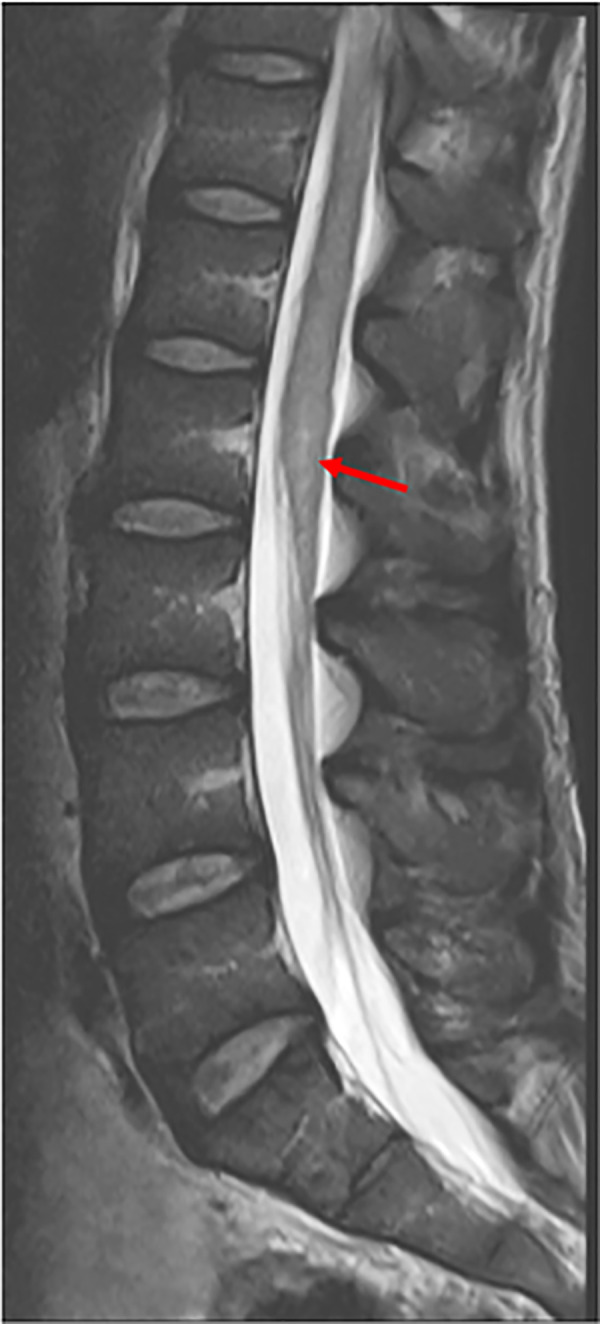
Initial MRI of patient 1 (locally reported as normal): showing subtle T2 hyperintense signal change (arrow) and mild swelling of conus medullaris.
